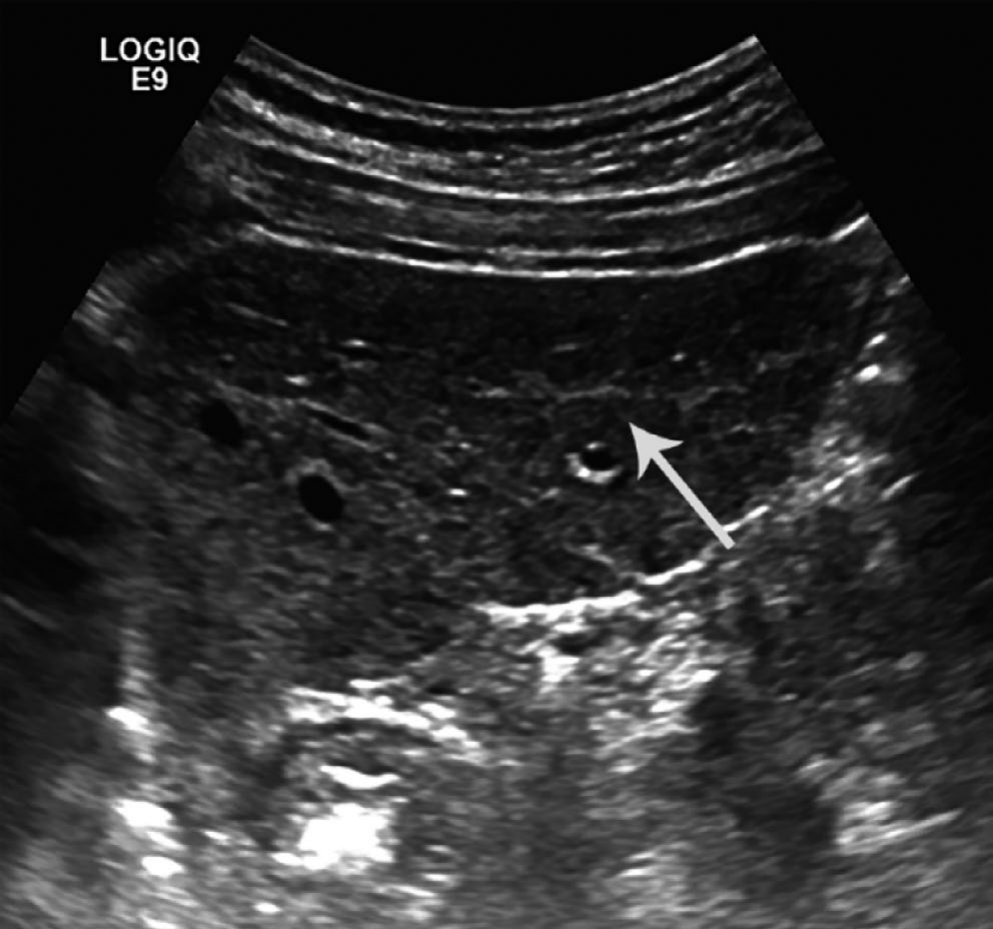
Hepatic fibrotic changes, Ultrasound
